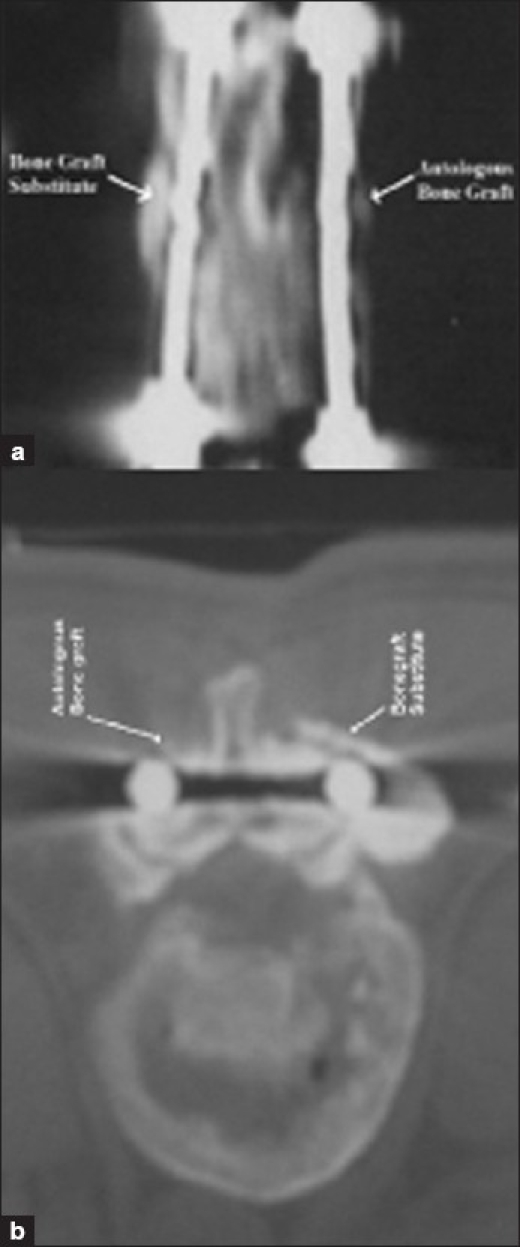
(a) CT scan of the same patient at one year followup showing evidence of good fusion on both sides. (b) CT scan axial cut of the same patient showing good fusion
